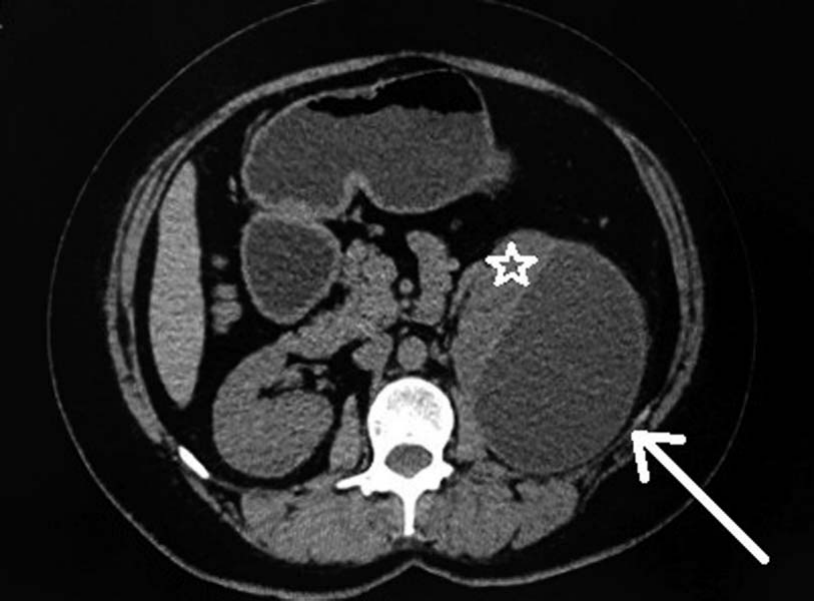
Axial noncontrast computed tomography shows hypodense collection in the subcapsular region of the left kidney (shown by arrowhead). The kidney appears compressed and displaced anteriorly (shown by a white star). The right kidney appears normal.
